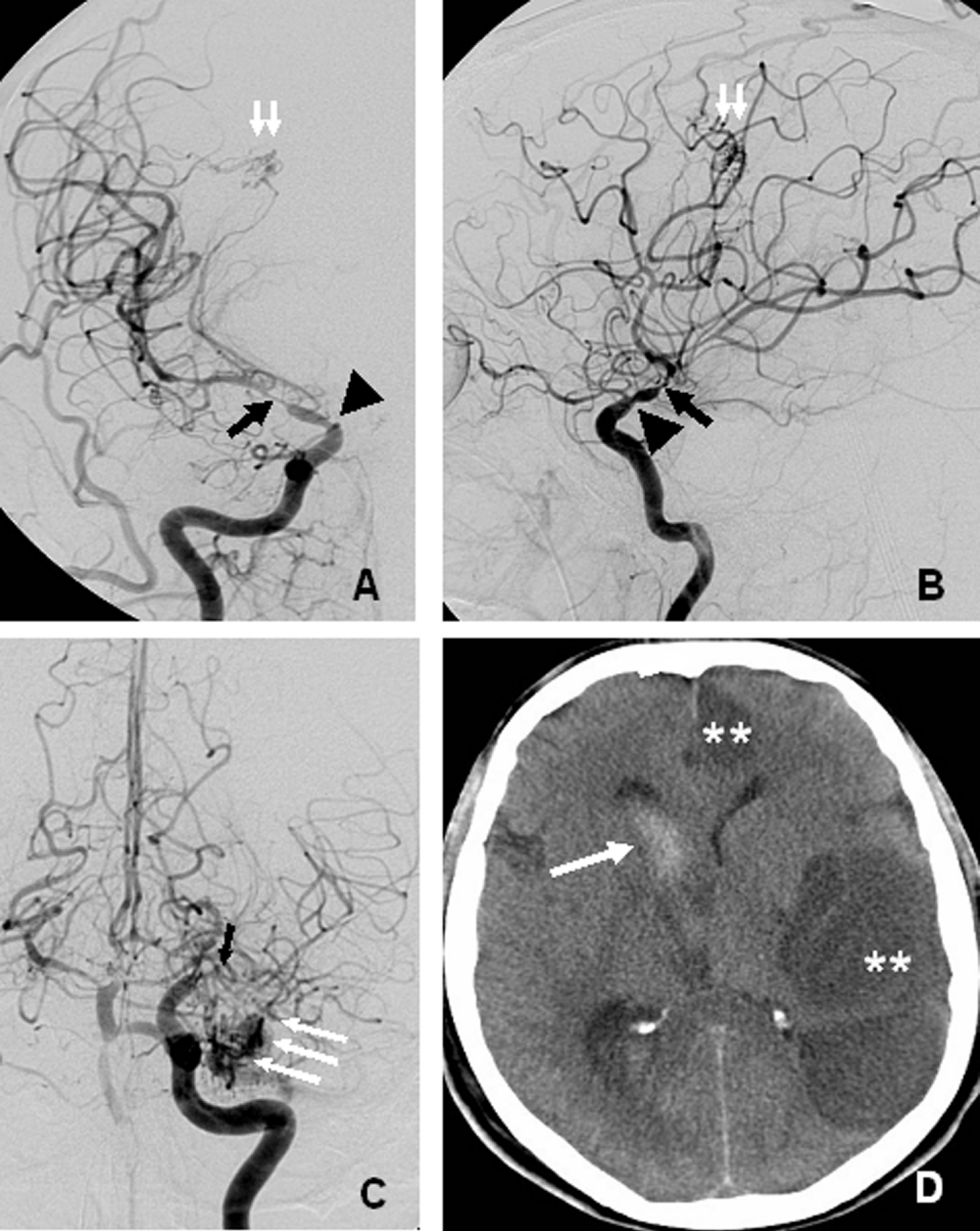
Cerebral digital subtraction angiography in a patient with vasospasm after rupture of a BAVM. Cerebral digital subtraction angiography performed 11 days after intraventricular hemorrhage in a 33-year-old patient with two brain arteriovenous malformations (BAVMs): a ruptured BAVM in the right frontal lobe and an intact BAVM in the left temporal lobe. Right internal carotid artery (ICA) injection, anteroposterior (AP) (A) and lateral (B) views: severe vasospasm of the M1 segment of the right middle cerebral artery (black arrow) and terminal right internal carotid artery (black arrowhead). Small frontal BAVM (double white arrow). Left ICA injection, AP view (C): severe vasospasm of the M1 segment (black arrow). The other BAVM is visible in the temporal lobe (triple white arrow). Cerebral CT scan (D): vasospasm-associated cerebral infarction in both the left middle and the left anterior cerebral arteries (). Note the remnant of the intraventricular hemorrhage (white arrow).
